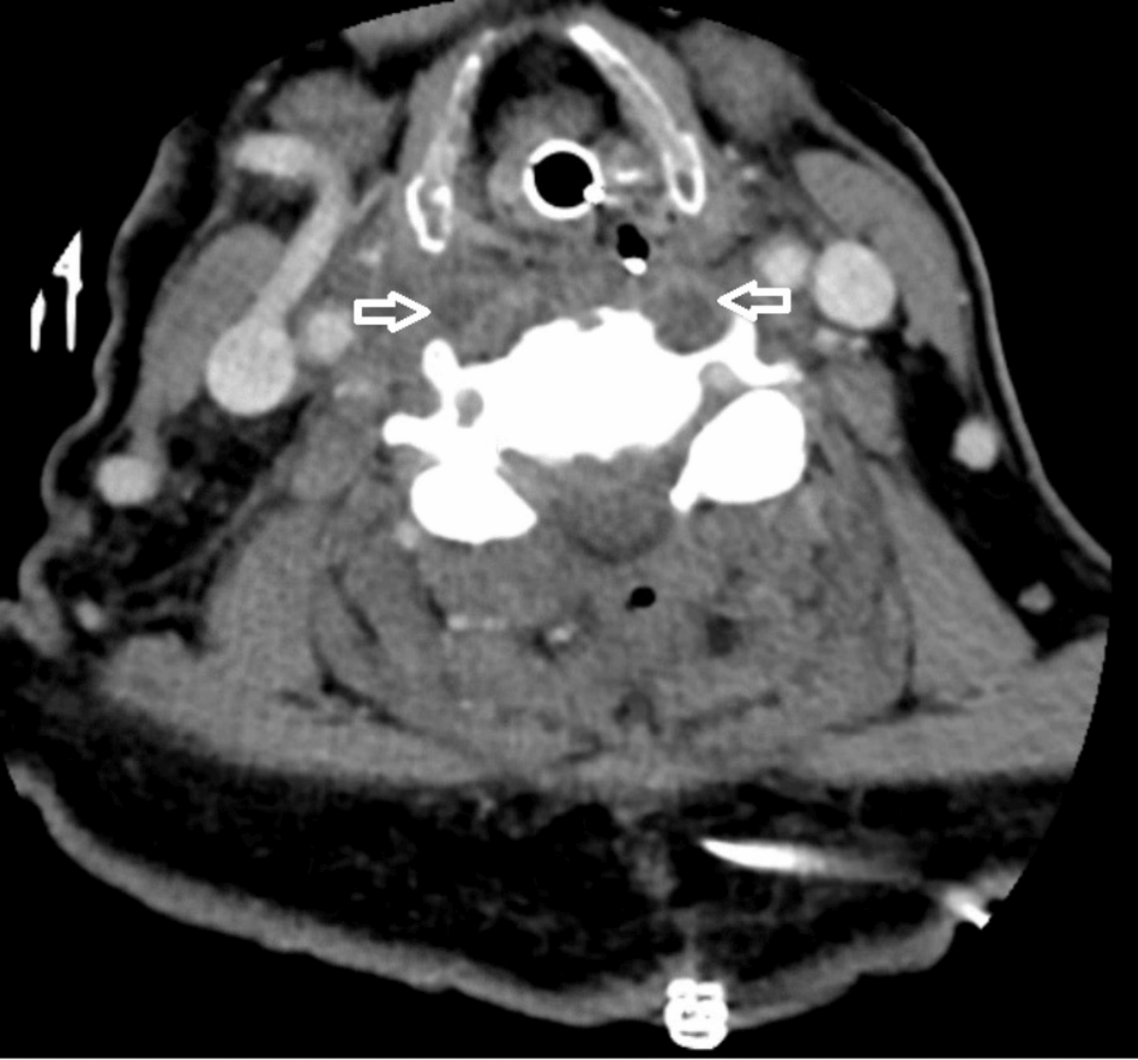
Cervical computed tomography showing pre-vertebral abscess (arrows).
